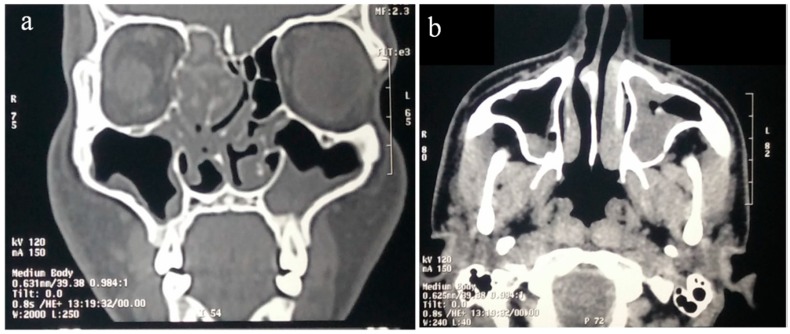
Coronal (a) and axial (b) computed tomography maxillofacial scan from the 18-year-old male patient with documented allergic fungal rhinosinusitis. There is opacification of left maxillary sinus and right ethmoid sinus with characteristic bony expansion and erosion.
